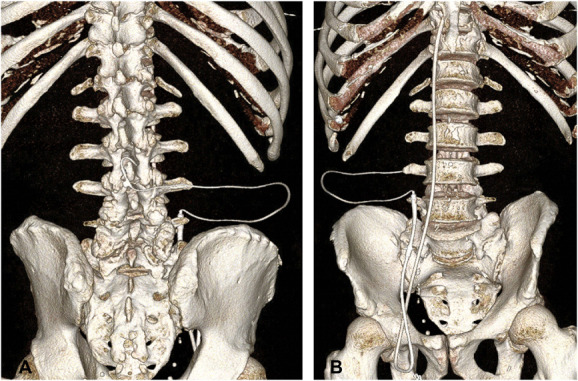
Computed tomography reconstruction with A, anteroposterior and B, posteroanterior views of the lumbocaval shunt.
